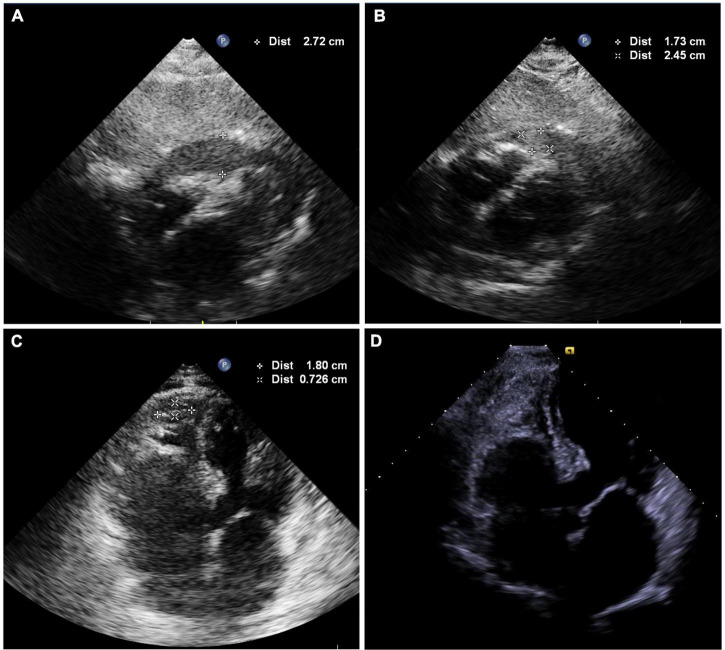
(A) Echocardiography showing hypoechoic (rather than anechoic) areas in the pericardial space. (B) Echocardiography shows mild effusions with a round hyperechoic thrombus in the vicinity of the right ventricular apex after intrapericardial thrombus aspiration. (C) Image showing shrinkage of the round thrombus, which appears as a strip in the vicinity of the right ventricular apex on the second postoperative day (Supplementary Video 8). (D) Echocardiography was obtained 2 weeks after discharge and showed no thrombus or pericardial effusion (Supplementary Video 9).
